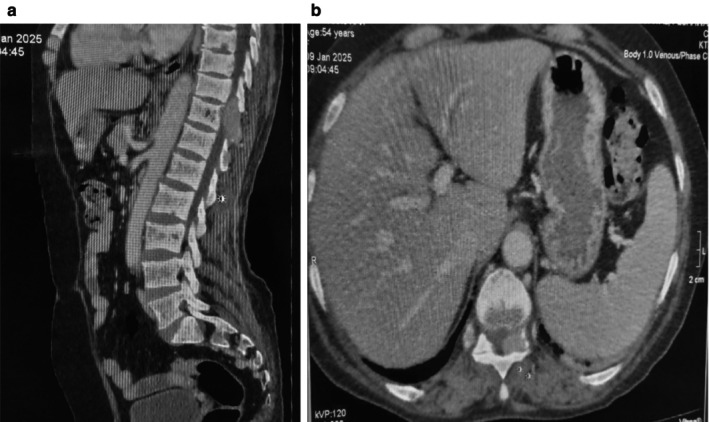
Sagittal and axial views of CT abdomen and pelvis showing osteolytic lesions at the D11/D12 level.
